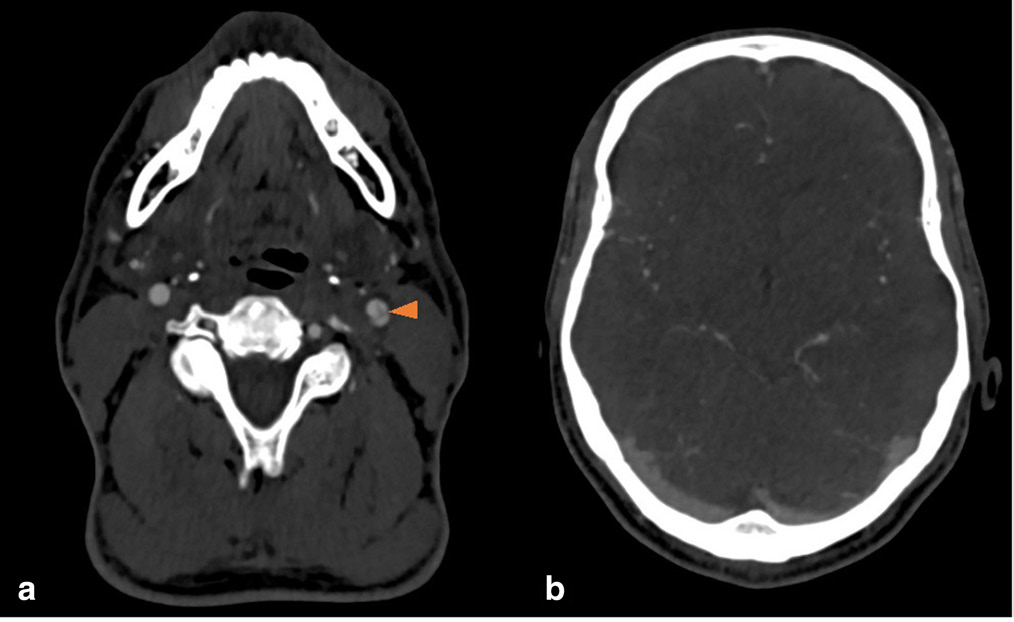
Follow-up axial CTA shows no residual clot in the left CCA and reveals the underlying intraluminal dissection flap shown by the orange arrowhead (a). Patent left MCA branches (b). CTA, CT angiography; MCA, middle cerebral artery.
